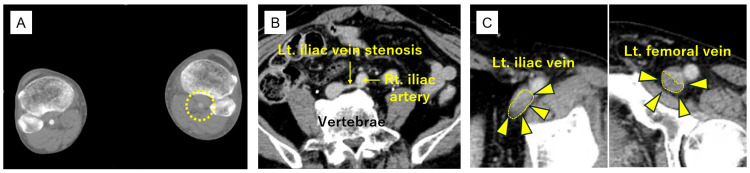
Preoperative computed tomography findings. (A) Contrast-enhanced computed tomography showing delayed opacification in distal arterial segments below the left popliteal artery, without clear occlusion (dashed circle). (B) Left iliac vein is compressed and stenosed between the common iliac artery and the lumbar vertebrae. (C) A filling defect consistent with thrombus (triangle and dashed area) extending from the left iliac vein to the femoral vein. Lt: left; Rt: right.
